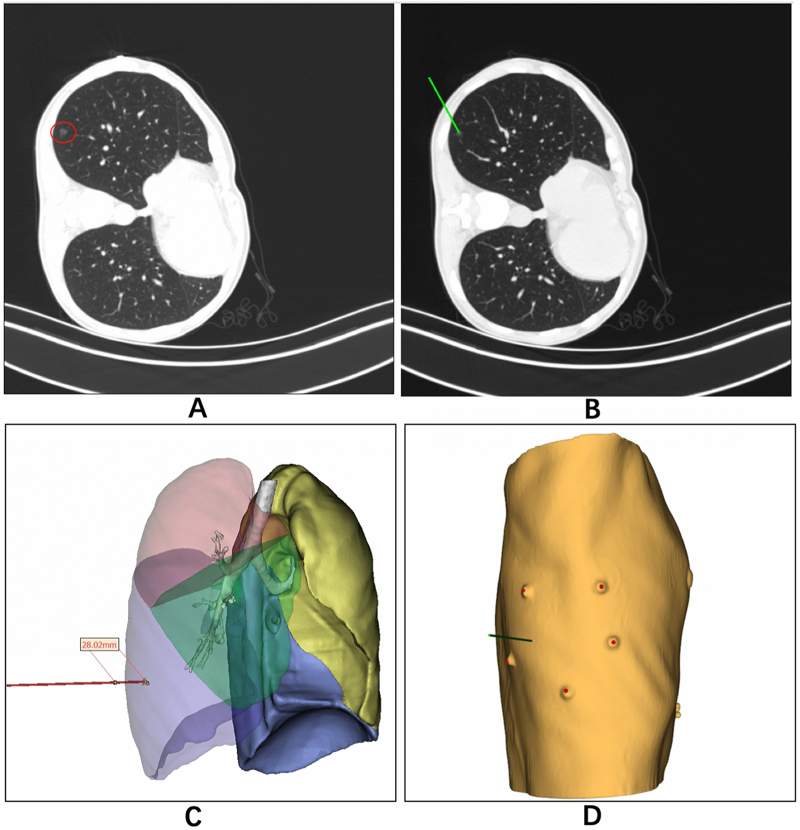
Preoperative CT and 3D planned path for the AR-guided PTLP for localizing lung nodule. The location of nodule (marked by the red cycle) was confirmed on the initial CT scan (A). Next, we planned the path for PTLP in the reconstruction software (the green line) (B), and a 3D digital twin model of the patient’s thorax with puncture path and depth was subsequently constructed (C). A model of five asymmetric labels on the chest wall for model registration and puncture guidance was also constructed (D).
